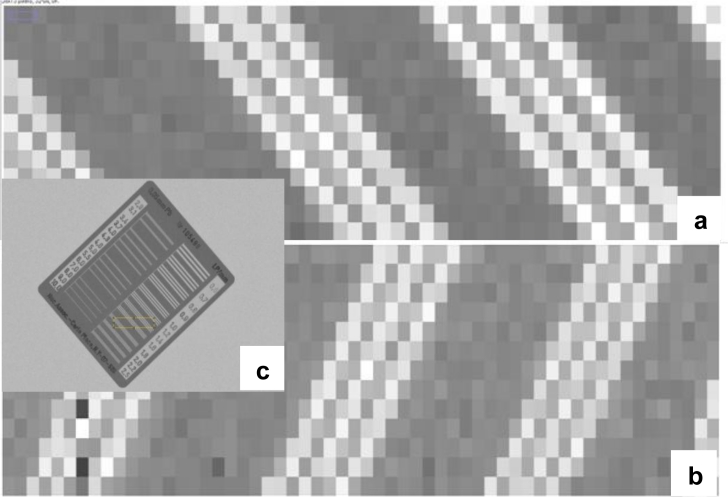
X-ray resolution image test from (a) a commercial FPD14 PPS array at 1.6 lp/mm shown in center of image (b) prototype 64 × 64 APS array at 2.0 lp/mm shown in center of image and (c) the resolution target.
